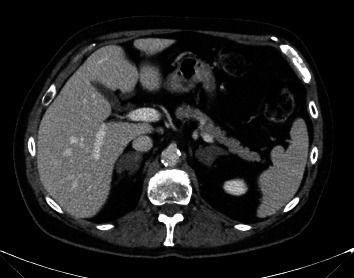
Preoperative CT scan was interpreted as benign bilateral adrenal adenomas measuring up to 3.9 cm on the right and 3.5 cm on the left, based on unenhanced attenuation <0 Hounsfield units and relative washout >40% on both sides.
